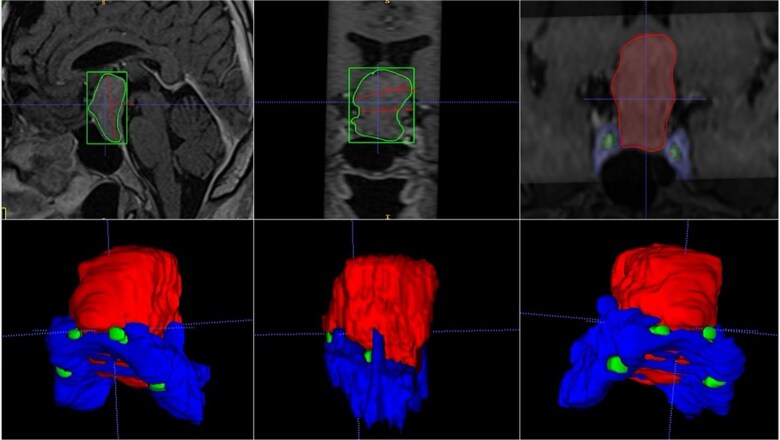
MRI and 3D volumetric segmentation of pituitary macroadenoma. Note the proximity of the internal carotid artery (green) in the most rostral and dorsal region of the tumor (red). Cavernous sinus (blue).
